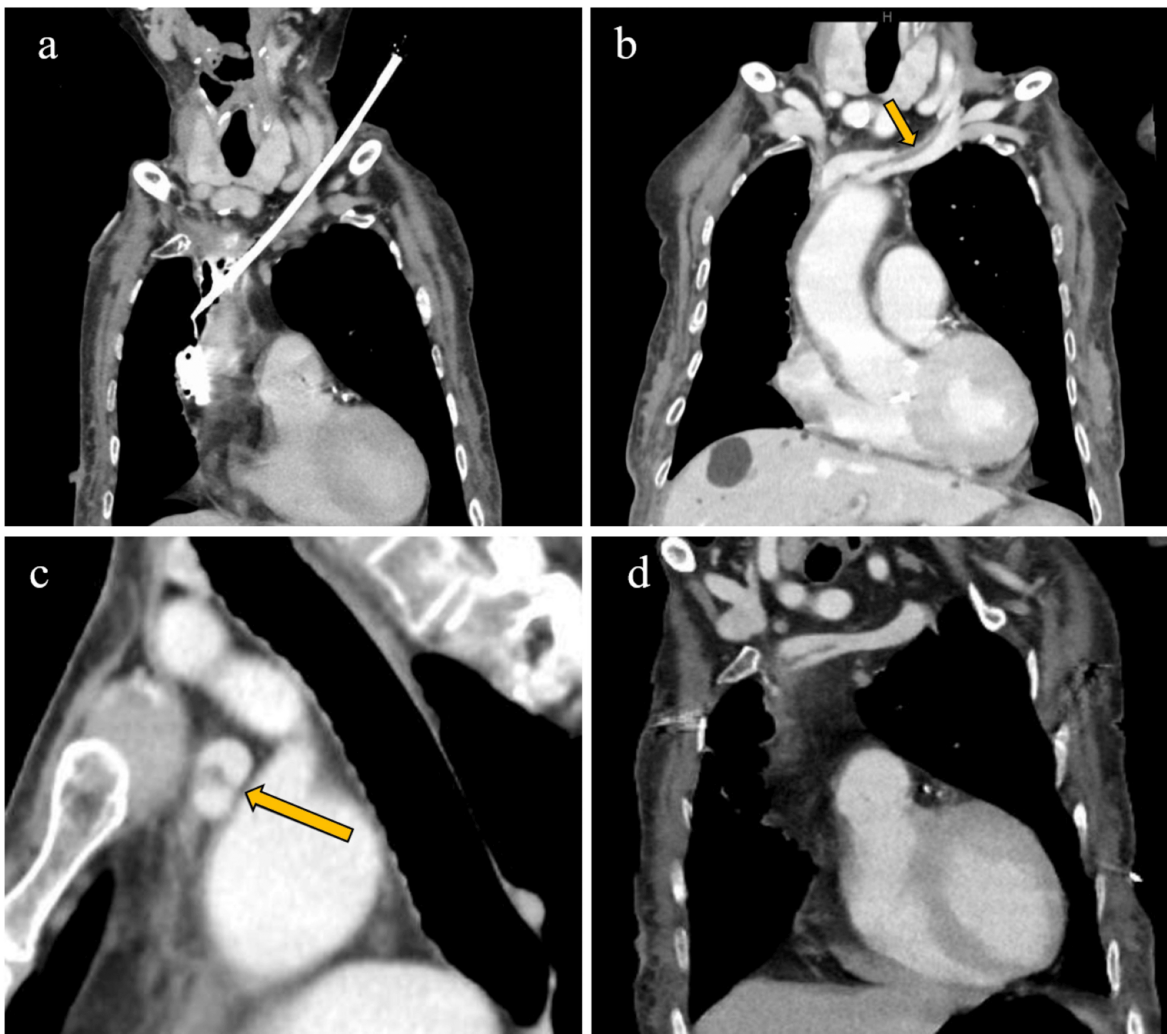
(a) CT showed the tip of the dilator outside the left brachiocephalic vein, and contrast medium had leaked into the mediastinum. (b) Contrast-enhanced CT showed the flap (arrow) and false lumen running from the proximal part of the left internal jugular vein to the brachiocephalic vein. (c) Sagittal reconstruction of contrast-enhanced CT (b) showed the flap (arrow) and false lumen in the left brachiocephalic vein. (d) Three weeks later, the flap remained, although some parts had become less distinct. CT: computed tomography
